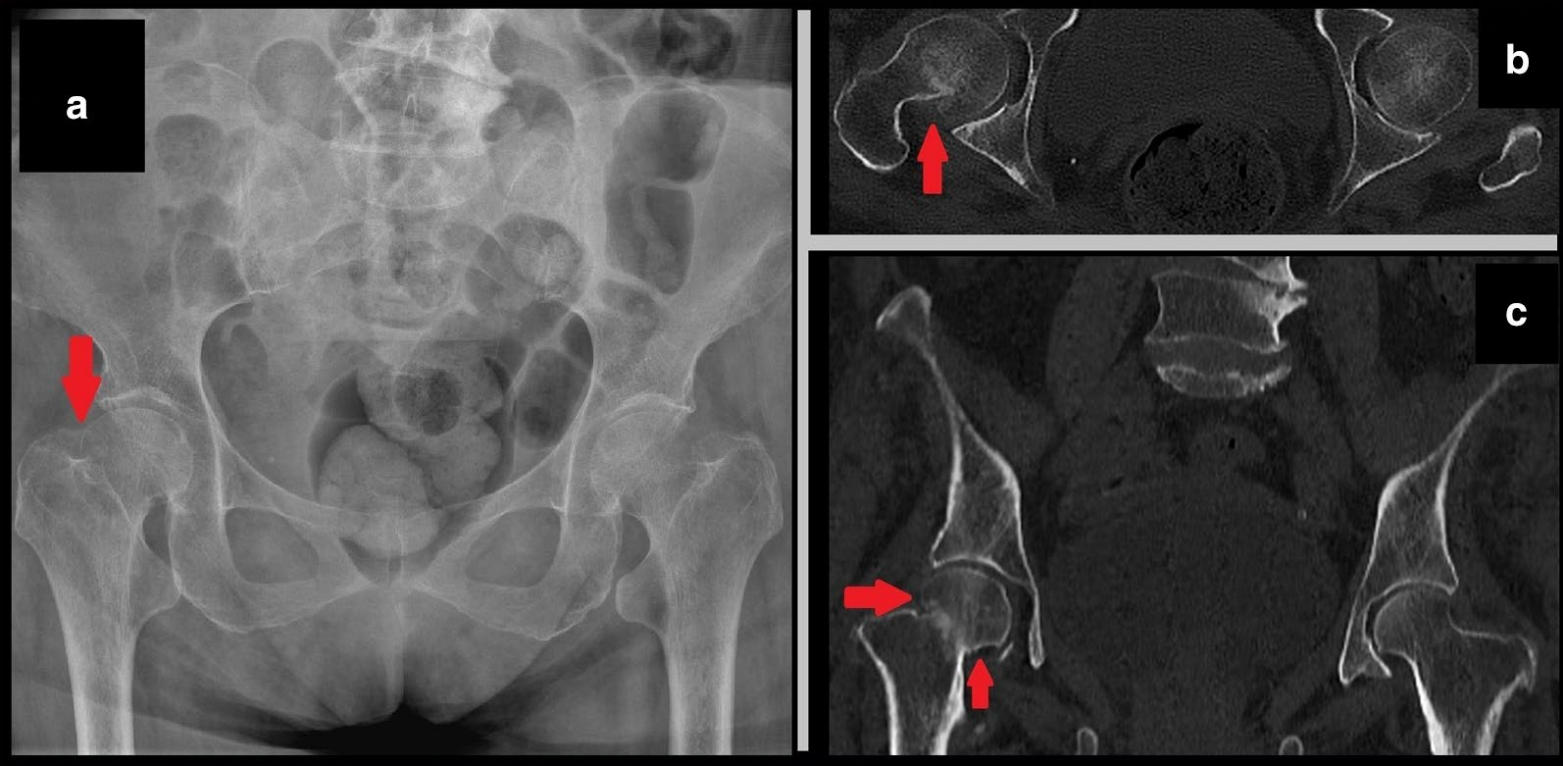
Under-reading (type 4) error with inattentional bias (Gorilla effect). A female patient was admitted to the emergency department with bilateral hip pain after falling down stairs. X-ray and pelvic CT were interpreted as “normal” by the on-call radiologist. However, shortening of the right femoral neck (red arrow, a) and a hyperdense line due to impacted fracture (red arrows, b, c) were missed. After retrospective detection of fracture, the radiologist was asked about the cause of missing these findings. He stated that he did not expect to see a hyperdense impaction fracture at all while evaluating that particular case. The prejudice of “fractures are seen as hypodense lines” caused inattentional blindness. A hyperdense fracture line is an uncommon finding, and due to its unexpected nature, may result in an under-reading error
